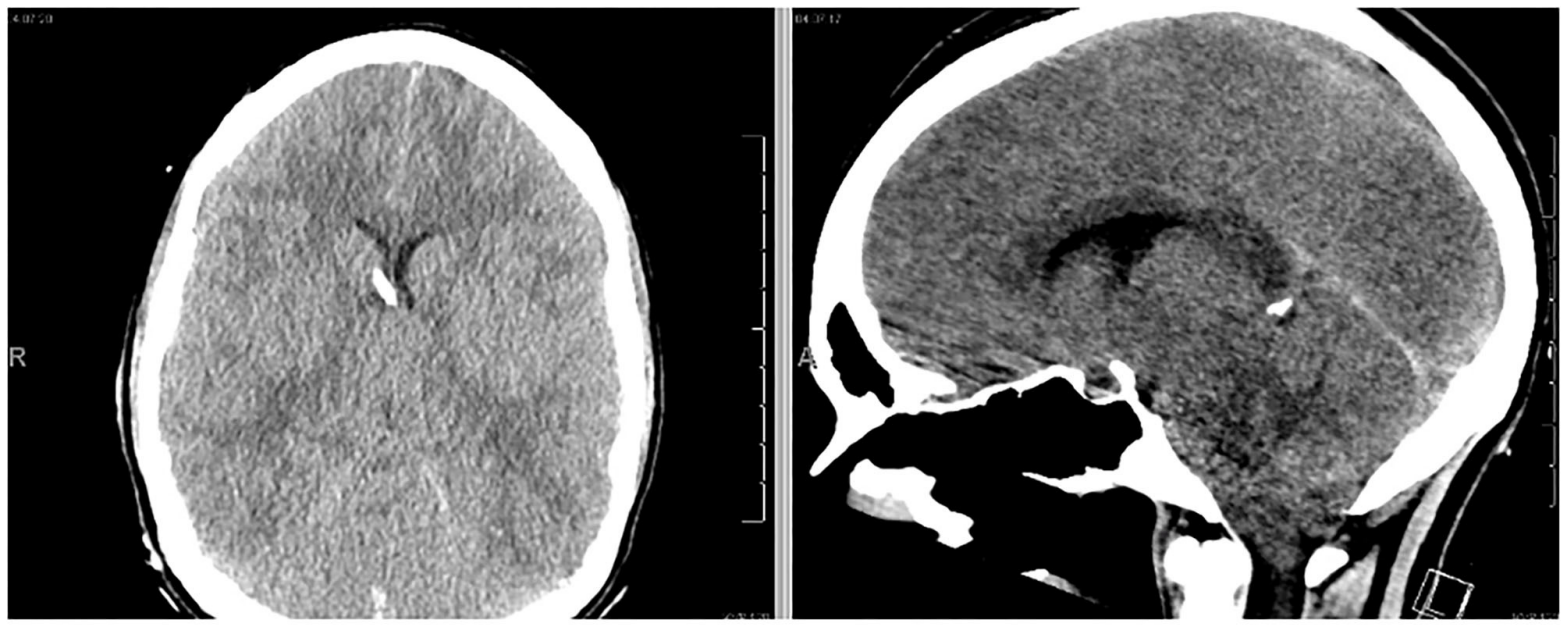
The patient exhibited anisocoria and severe headache following hospital admission. Follow-up CT scan demonstrated profound cerebral edema and downward tonsillar herniation. An EVD was successfully placed (left image).
